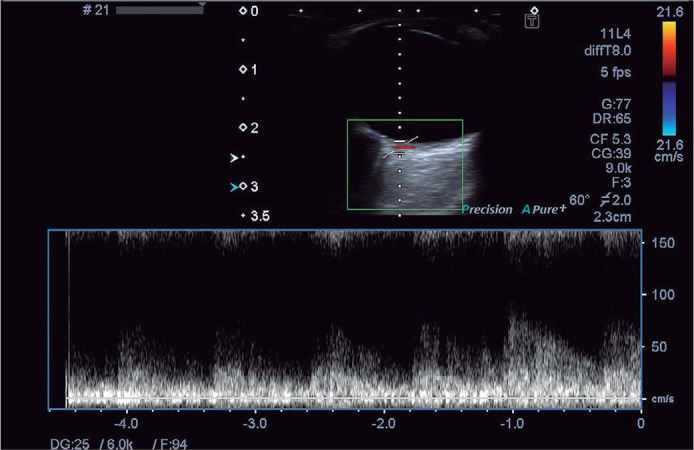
Doppler ultrasound images of posterior ciliary artery.
